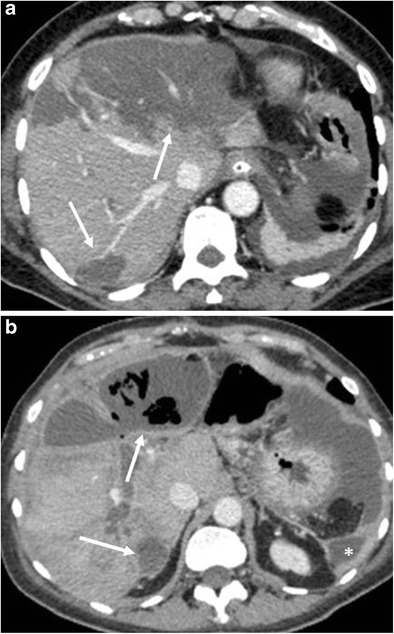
Hepatic infarction. a CT image shows multiple hypodense and hypovascular areas of infarction (white arrows) following a pancreaticoduodenectomy. b CT image 4 weeks later in the same patient. The areas of infarction show a thickened and enhancing wall with multiple air bubbles within (white arrows), findings consistent with hepatic abscesses. Another abscess is visible at the level of the left lateroconal fascia (*)
